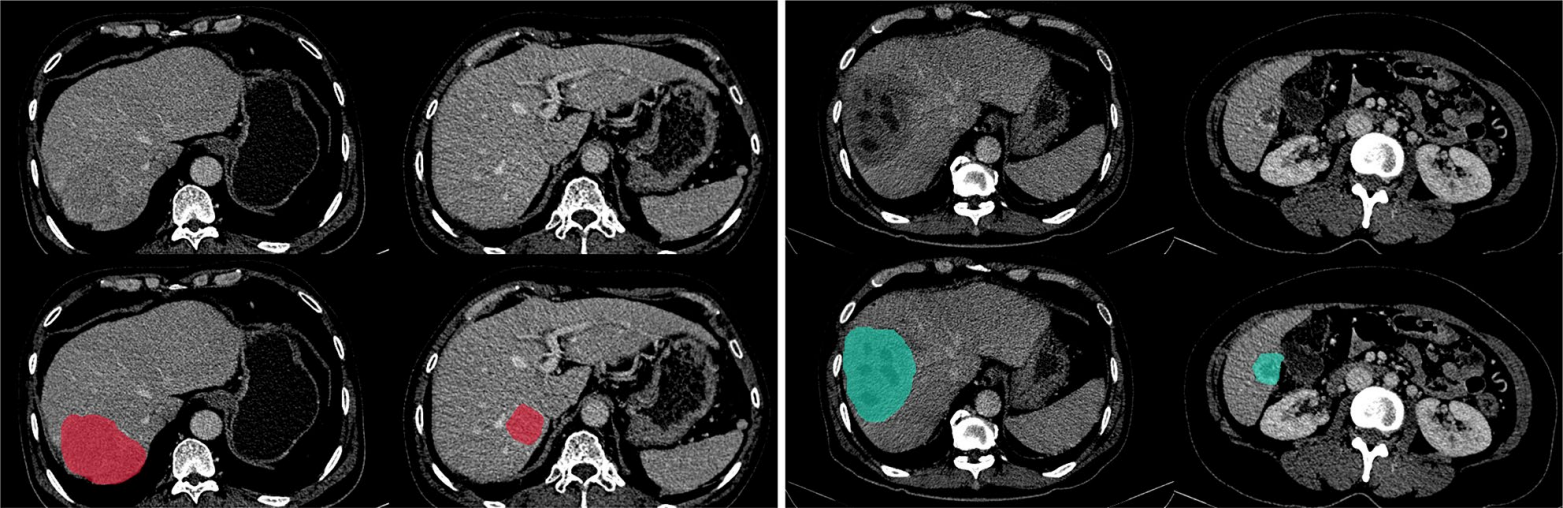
Examples of CT venous slices (top row) and the hepatic lesion annotation overlaid (bottom row) in out dataset. HCC tumors (red) are in the first two CT slices, and non-HCC lesions (cyan) are in the last two CT slices. Best view in color.
